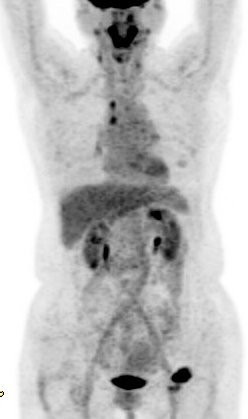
Fluorodeoxyglucose (FDG) positron emission tomographic scan showing areas of FDG accumulation in the mediastinum and left inguinal region.
